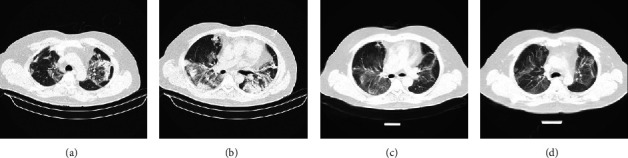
A 51-year-old man with COVID-19 pneumonia with a 17/25 severity score receiving remdesivir and dexamethasone for treatment. (a, b) The axial chest CT scan depicts air space opacities with predominant consolidation. (c, d) Chest CT 8 weeks after hospital discharge demonstrates residual faint ground-glass opacities and subsegmental atelectasis.
